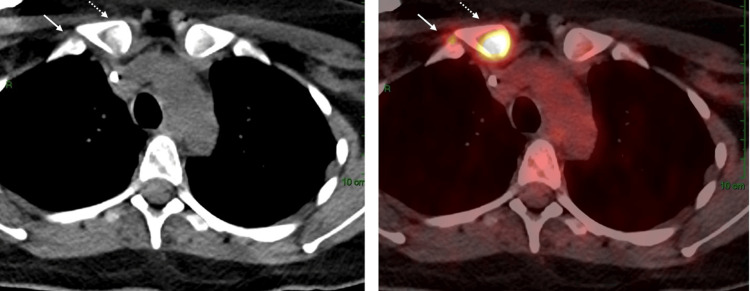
A PET-CT scan shows a new focus of uptake in the right first rib contiguous to the right clavicle.
